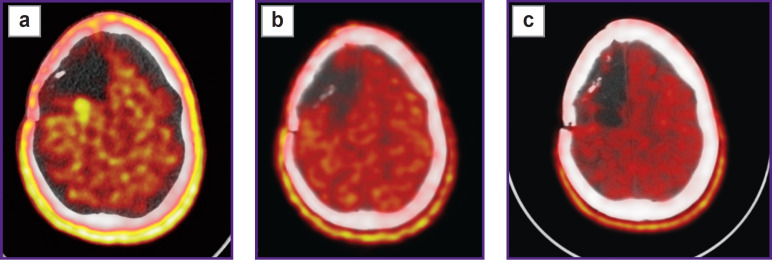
PET/CT with [^11^C] methionine: (a) a radiopharmaceutical agent accumulation area is determined along the posterior contour of the postoperative cyst (accumulation index — 2.4) with the dimensions of 13×24×17 mm; (b) 1 month after stereotactic photodynamic therapy; (c) 9 months after stereotactic photodynamic therapy, no relapse
